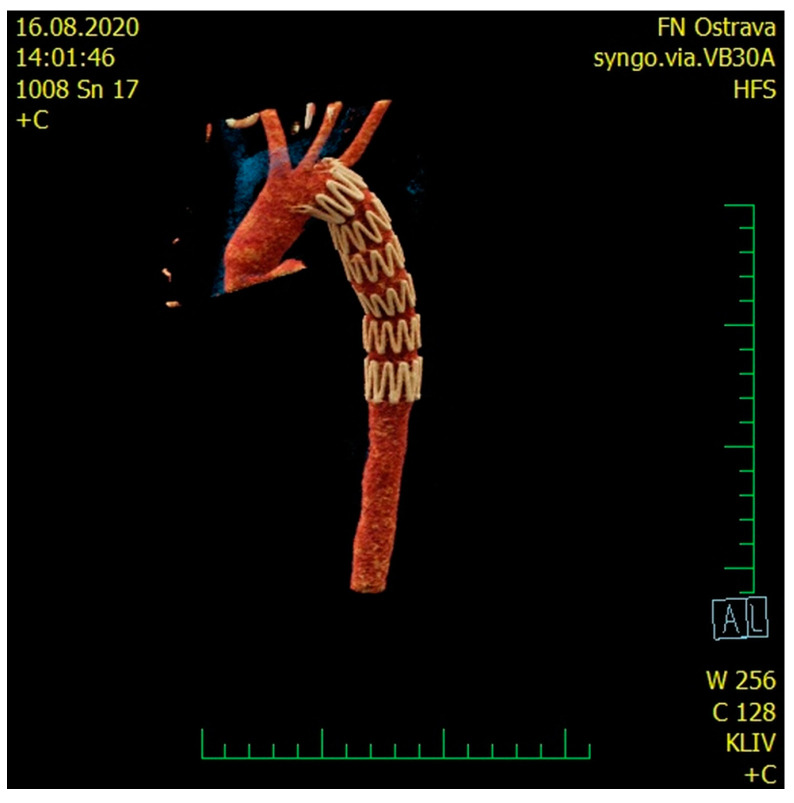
CT 3D reconstruction of the aortic arch with the implanted aortic stent-graft.
